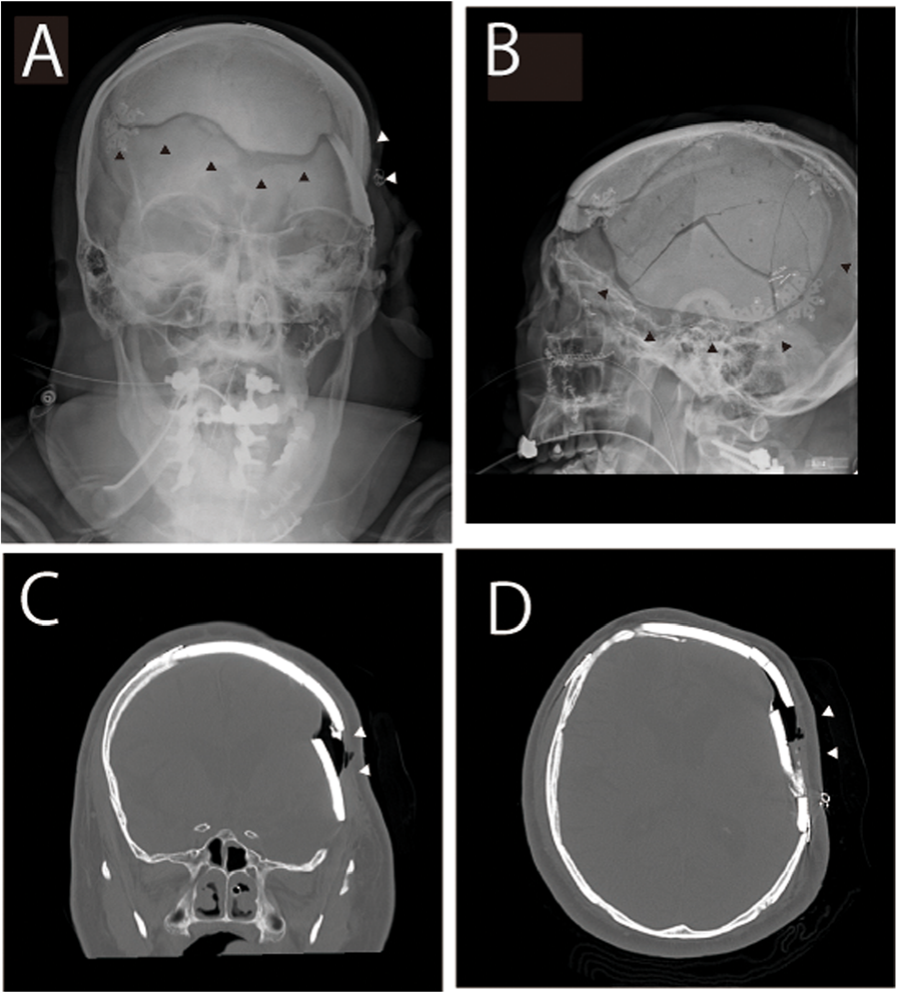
Skull X-ray and CT images. a Coronal view and b sagittal view X-ray image showing dislodgement of the left temporal region of the skull (white arrows) and the artificial cranial bones (black arrows) loosely fixed to the patient’s skull. c Coronal view and d transverse view CT image showing dislodgement of the skull (white allows)
